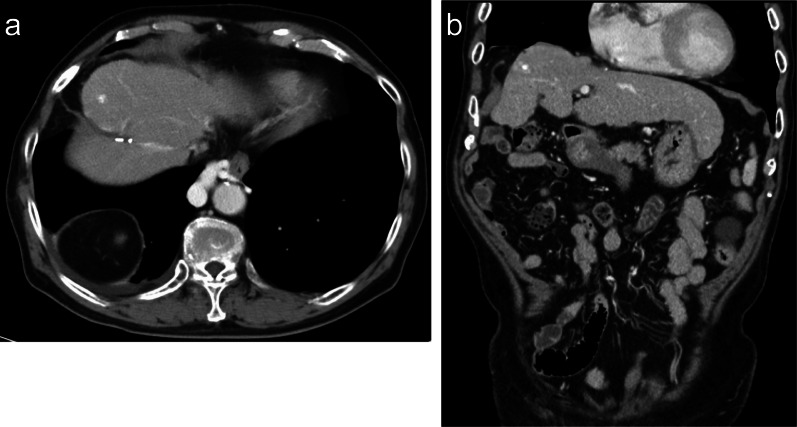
CT image half a year before emergency surgery. a Axial view, b coronal view. There were no findings of diaphragmatic hernia or Chilaiditi syndrome
